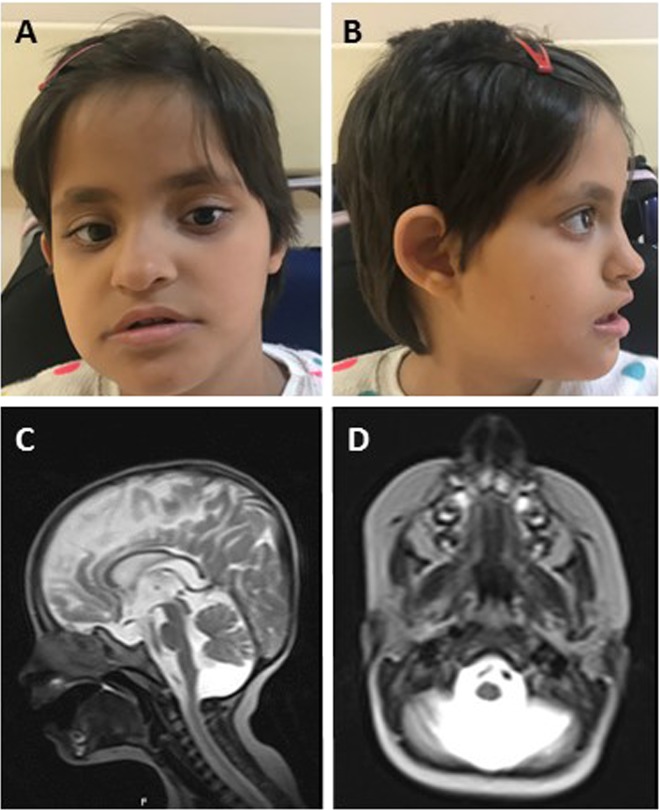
Clinical findings. a, b Patient photographs at 6 years of age showing facial dysmorphism: telecanthus and upslanting palpebral fissures, thin vermilion upper lip, big mouth and broad nasal bridge; b Sagittal T2-weighted cranial MR images showing cerebellar vermis hypoplasia (Dandy-Walker variant); c Axial T2-weighted cranial MR images showing cystic dilatation of the cerebellum indicating cerebellar vermis hypoplasia
